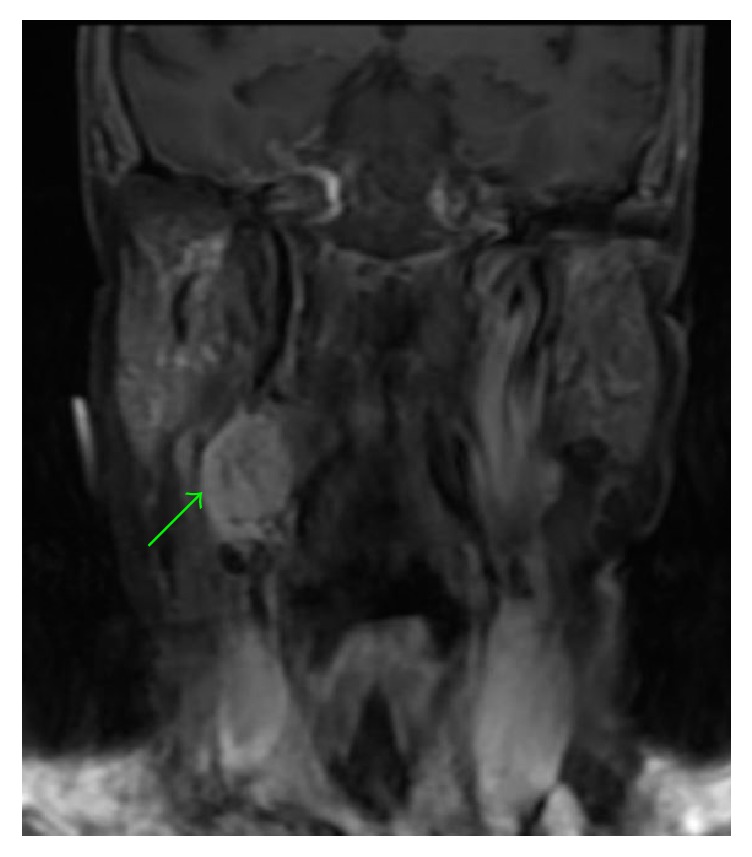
MRI, coronal section, T1-weighted with fat saturation and contrast. The green arrow refers to the neck mass.
